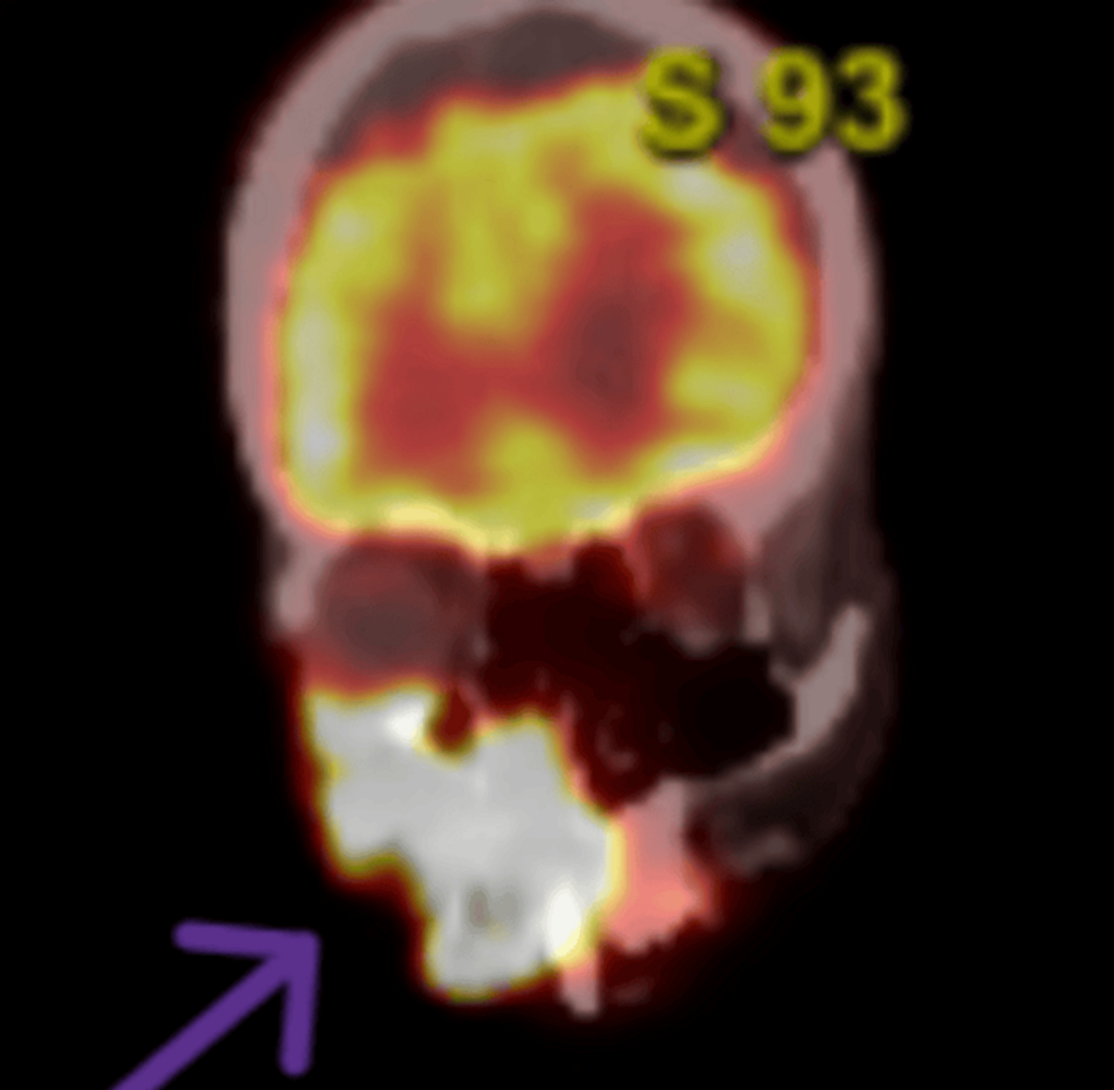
Coronal PET-CT image showing FDG-avid right facial tumor. PET-CT in the coronal view revealed a markedly fluorodeoxyglucose (FDG)-avid infiltrative mass in the right midface, consistent with aggressive basal cell carcinoma involving soft tissue and underlying bone.
